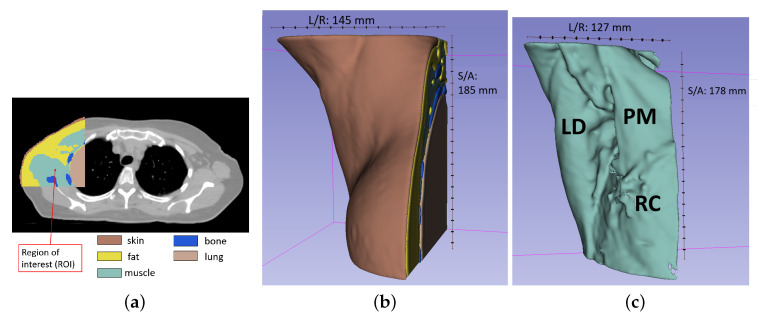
(a) Axial view of the body at the level of the sternum: the segmented organs are superimposed to the original Computed Tomography (CT). Each tissue is represented by a different color (legend). The red arrow points at the segmented region of interest. (b) 3D-rendering view in 3D-slicer of the segmented organs. (c) 3D-rendering view in 3D-slicer of the segmented muscle tissue. Different muscles of the region are indicated: pectoralis major (PM), latissimum dorsi (LD), and rib-cage (RC) muscle. Superior/Anterior (S/A) and Left/Right (L/R) dimensions of the model are reported.
